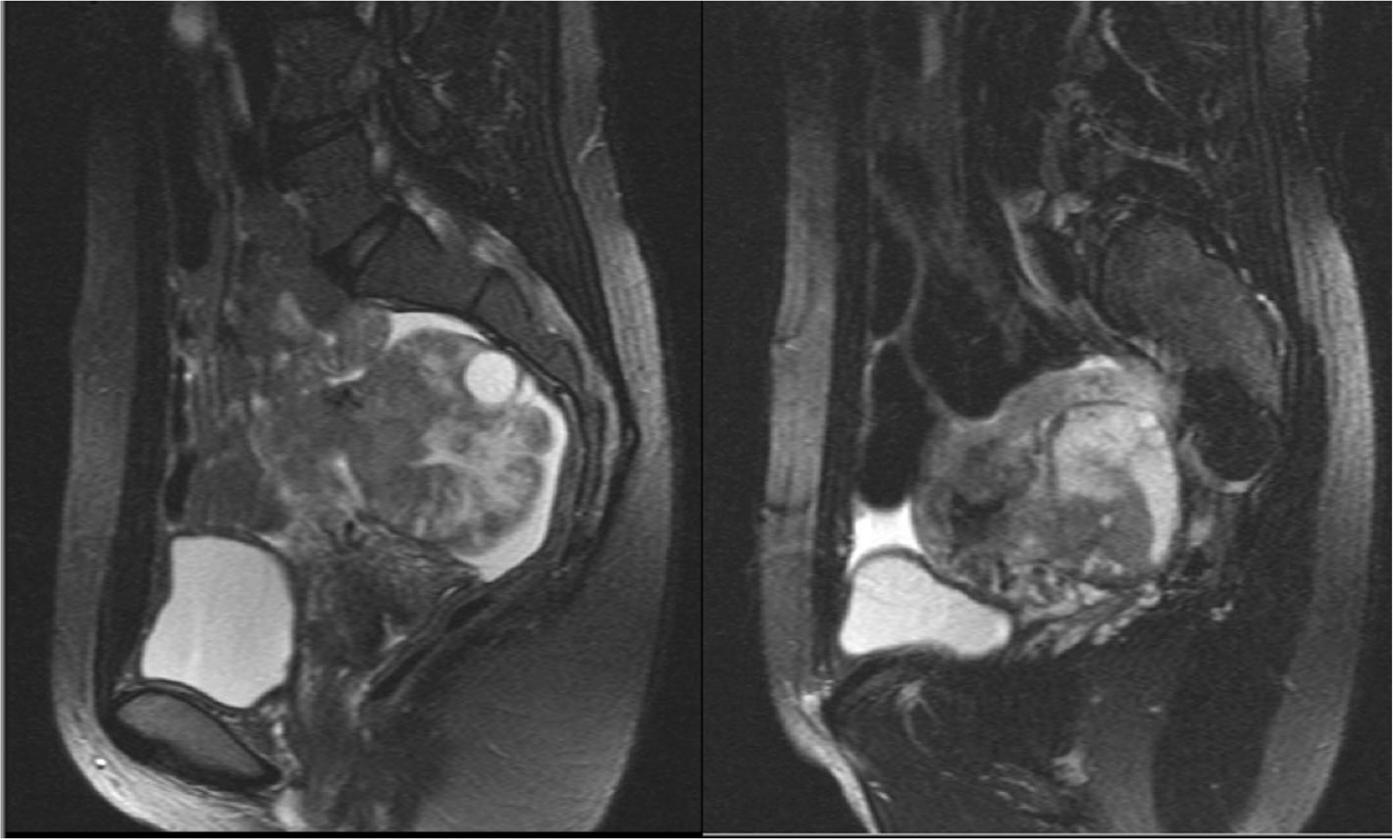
Magnetic resonance imaging of the pelvis. Magnetic resonance imaging showed an inhomogeneous tumor mass in the lesser pelvis with a size of 11.4 × 8.6 × 11.7 cm
